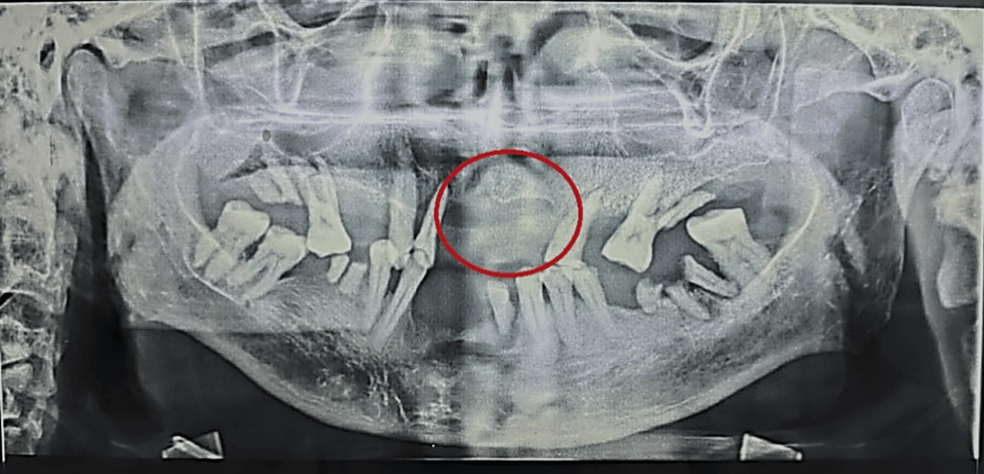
Orthopantomogram showing bone loss with foci of calcifications
